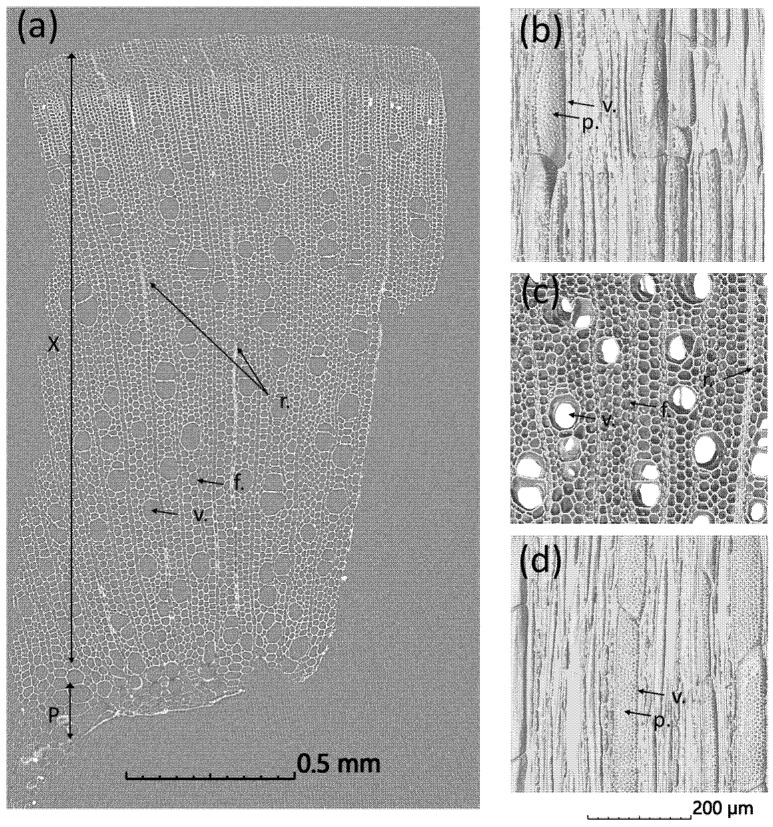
X-ray nanotomography image of the transverse cross-section of a hemp SHI-W sample showing the different tissues and cell types (a) and X-ray computed nanotomography images of a sample in L-R (b), R-T (c) and L-T (d) planes (X: xylem, P: pith, v.: vessel, f.: fiber, r.: ray and p.: pit).
